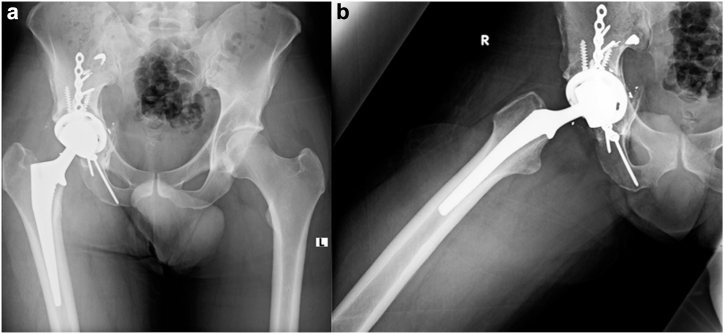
(a) AP and (b) axial radiographs taken at the 6-month follow-up of the right femur and acetabulum, demonstrating complete fracture healing and proper orientation of the THA components. AP, anteroposterior; THA, total hip arthroplasty.
